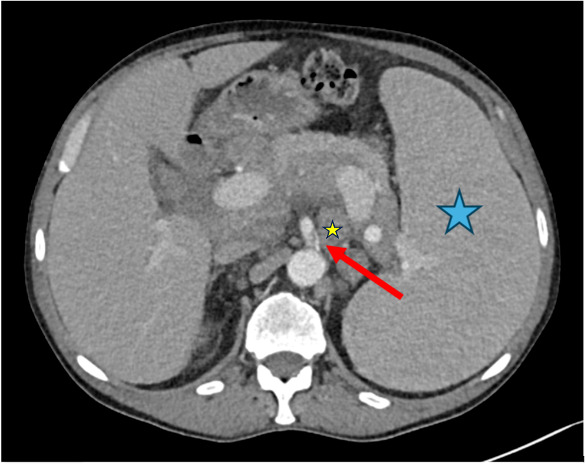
Case 4, 66-year-old male with history of marginal zone lymphoma. A chest CT scan was performed after recovering from COVID-19 to assess post-primary changes in the lung. No pathological lung findings were observed. However, an incidental finding of a short dissection in the superior mesenteric artery was noted (red arrow). Additionally, the spleen (blue asterisk) and retroperitoneal lymph nodes (small yellow asterisk) are enlarged, consistent with the underlying disease.
